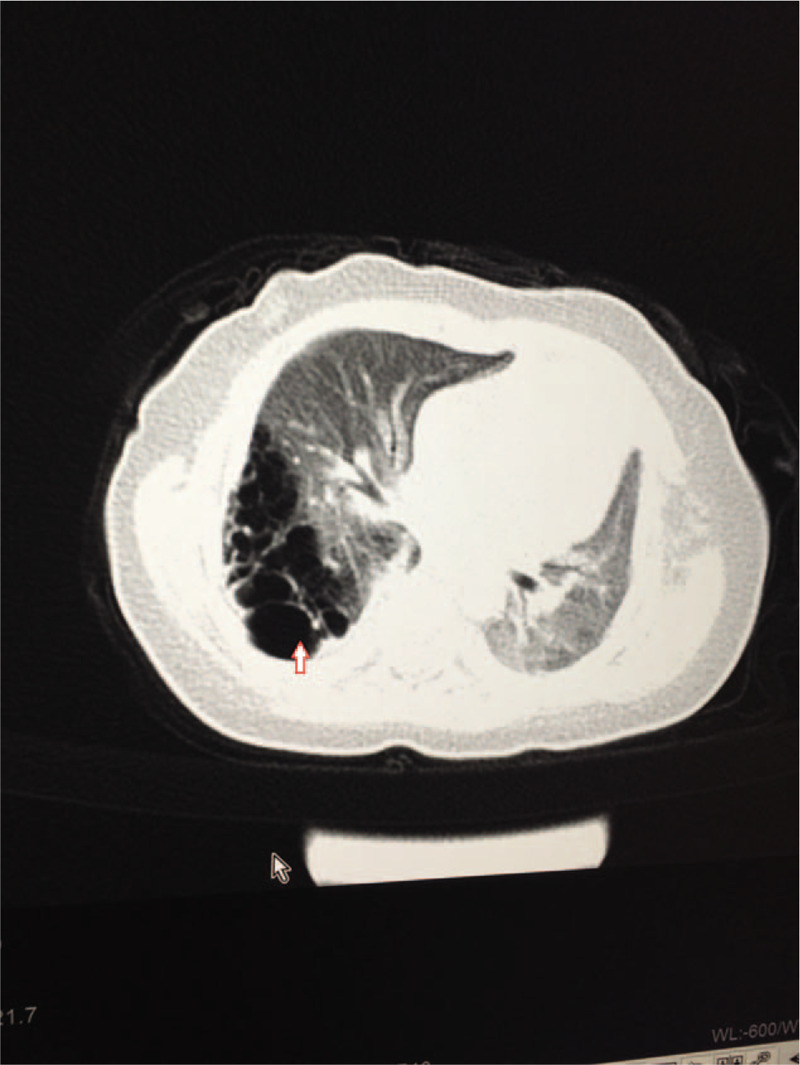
Chest CT examination at cross section of eighth thoracic vertebra-position after hospitalization. CT examination showed an increased volume in the right lung (especially at the lower lobe) with a large soft tissue density shadow at the lower leaf, non-uniform internal density without any bronchial air sign, and a cystic low-density shadow (arrowed) with clustered distribution of thick uneven wall. Mediastinum leftward shift to compress the left lung.
